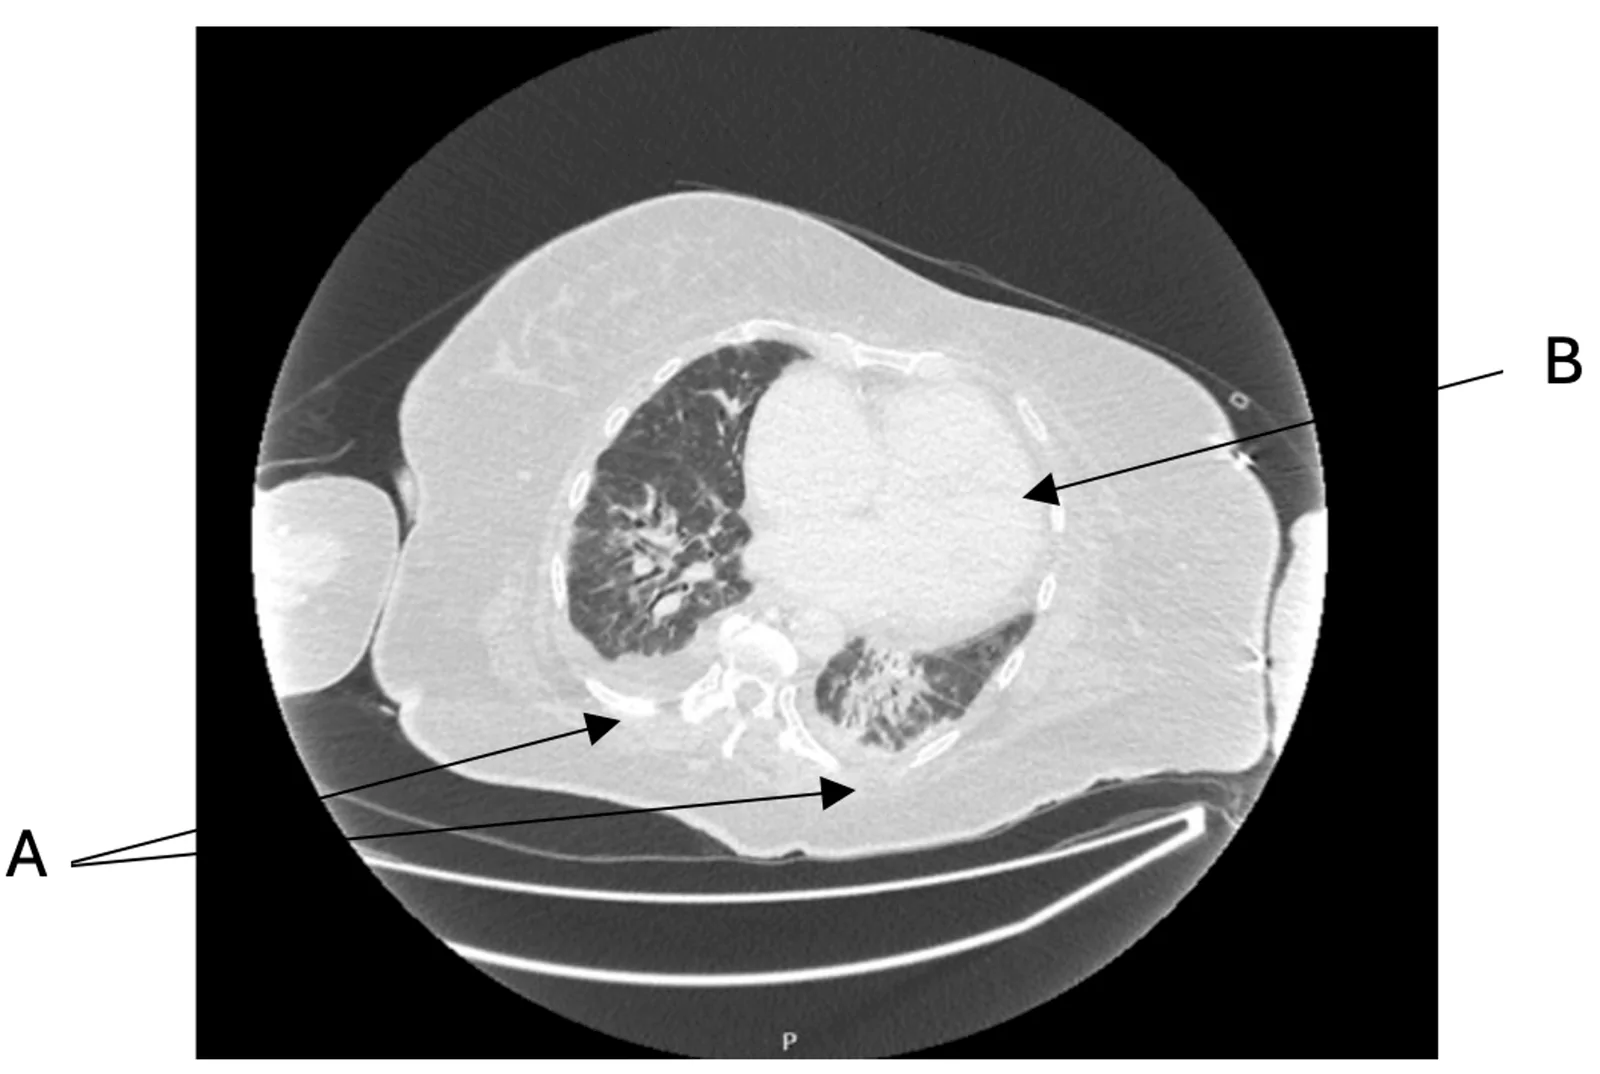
Axial Computed Tomography Image of the Thorax Axial computed tomography imaging of the thorax demonstrating bilateral pleural effusions (labelled A) and a small pericardial effusion (labelled B), with minor areas of ground-glass attenuation.
